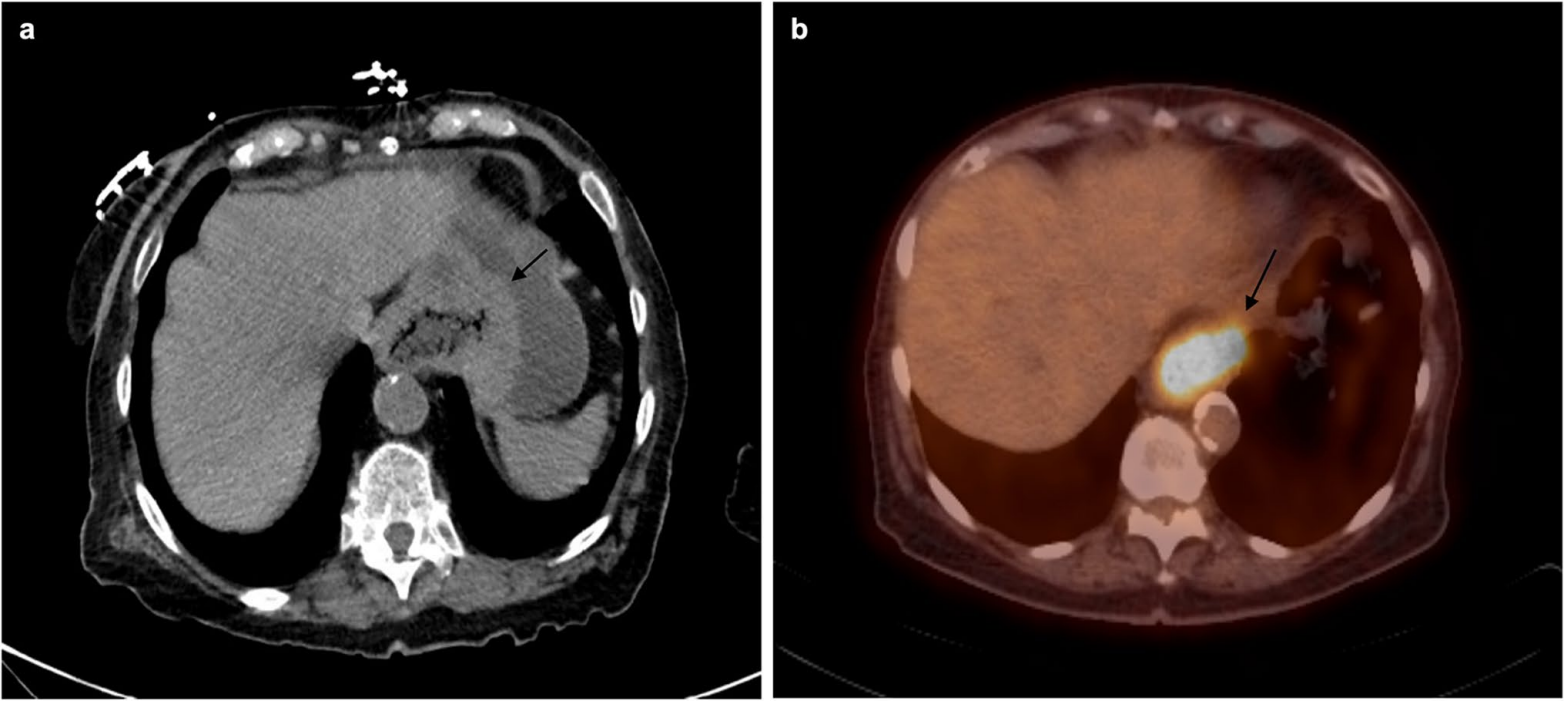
Axial CT scan (a) demonstrating an infiltrative circumferential mass in the distal esophagus extending into the proximal stomach (arrow) that disrupts the normal smooth curvature of the stomach. The following steps include confirmation and further evaluation via endoscopy. PET/CT F-fluorodeoxyglucose (b) avidity associated with lower esophageal wall thickening extending into the GE junction and gastric cardia (arrow) corresponding to known GE malignancy
